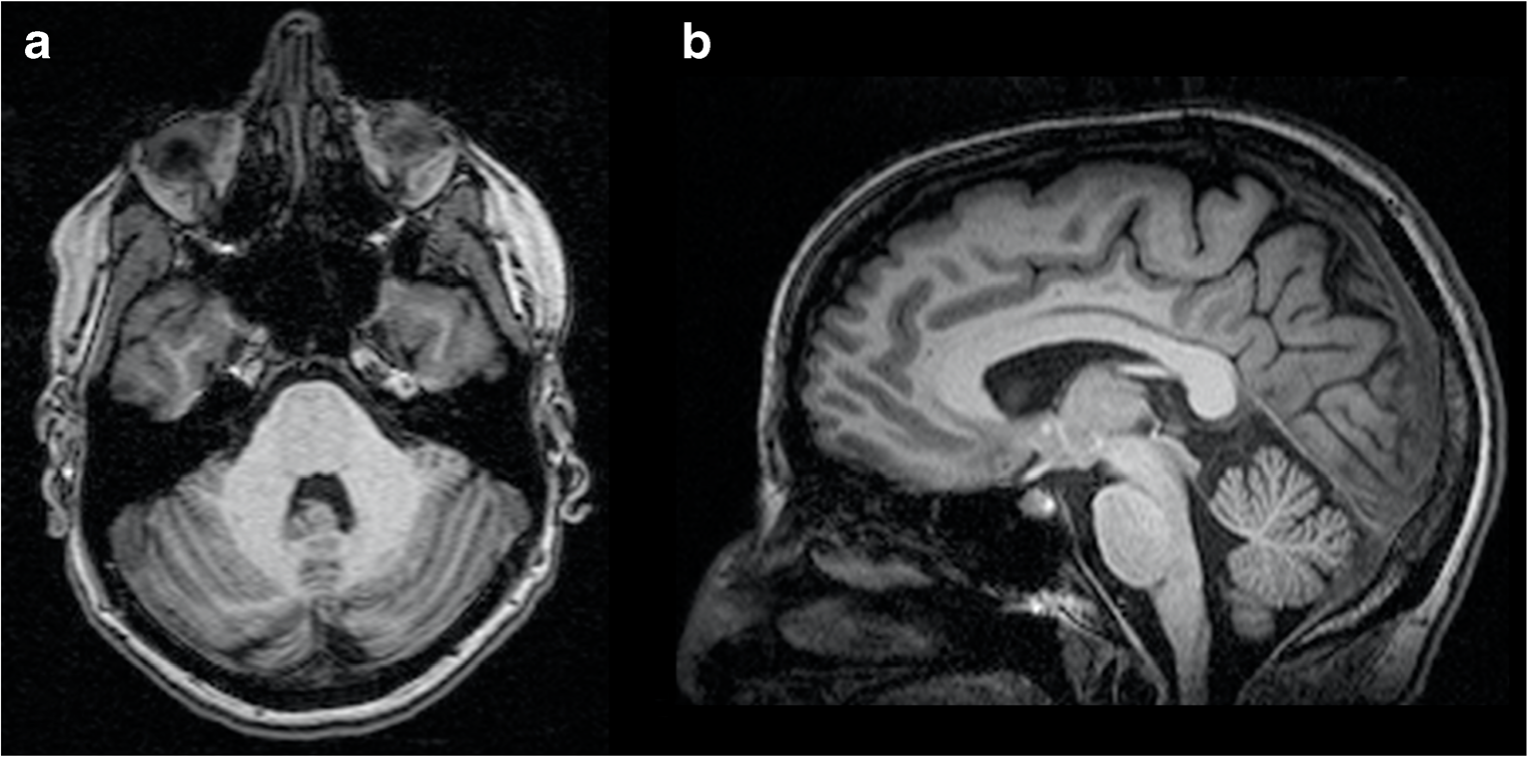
Axial (a) and sagittal (b) multiplanar reconstructions of a 3D T1-weighted volume show the typical brain MRI appearance in FRDA patients, as depicted in this 20-year-old male subject where a preserved cerebellar volume is present
